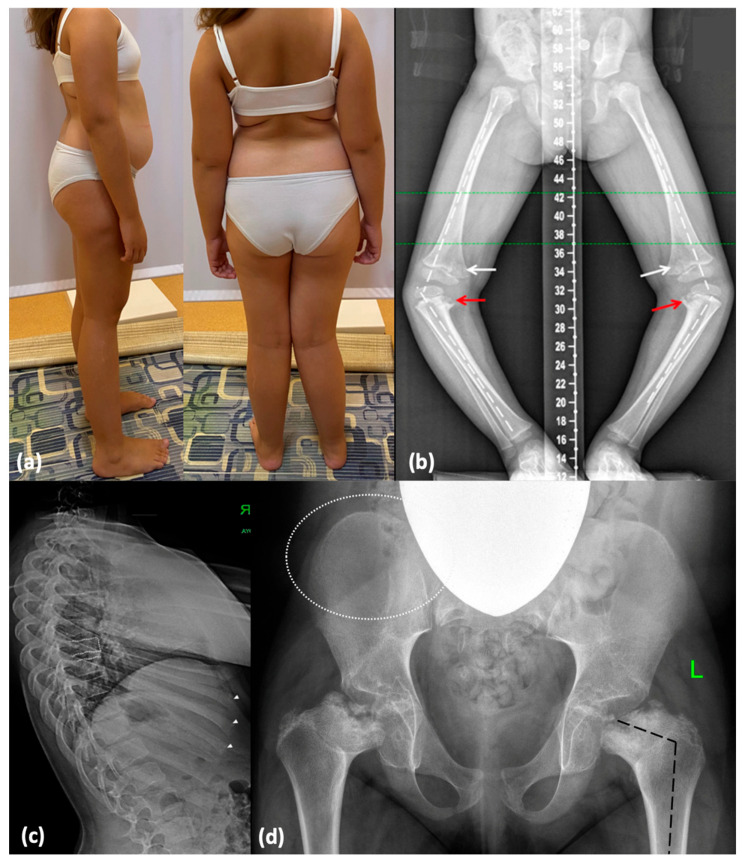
Phenotype and skeletal radiographies of P2 at various ages: (a) A photo taken at 9 years and 4 months. (b) Severe varus deformity (white broken lines) secondary to the Blount-like tibia vara (red arrows) and metaphyseal irregularities predominantly in the medial parts of the femur (white arrows) (3 y.o., before surgery). (c) Irregularities of the vertebral endplates are mildly represented (white dotted lines), and distal parts of the ribs are mildly enlarged (white arrowheads). (d) Coxa vara (black broken lines) and abnormal ossification of the femoral neck and head; minimal lace-like appearance of the iliac crests (indicated by white dotted lines).
